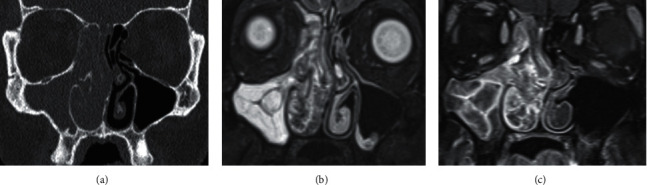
Inverted papilloma (IP) in a 32-year-old male with a 18-month history of rhinorrhoea. (a) Coronal unenhanced CT shows right unilateral maxillary sinus opacification (UMSO) with complete obstruction of the ostiomeatal unit. Bowing of the nasal septum and amorphous faint calcification could be associated with fungal disease; however, (b) coronal STIR and (c) contrast-enhanced T1W demonstrate a right nasal cavity lesion with the typical “cerebriform” appearance of IP and attachment point at the inferior orbit. Hyperintense right UMSO with mucosal enhancement indicates inspissated secretions rather than lesion extension.
